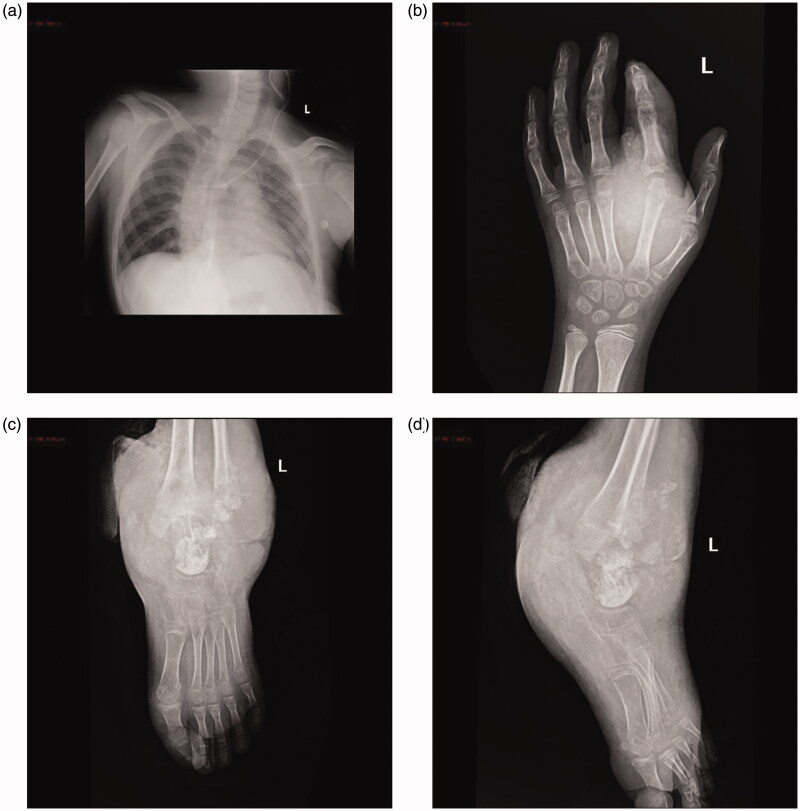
(a) Chest radiography showing thoracic scoliosis (anteroposterior and supine views). (b) X-ray image of the left wrist showing a soft tissue mass adjacent to the metacarpal and proximal to the second finger. (c) X-ray image of the left ankle showing a large soft tissue mass with intramass calcification.
